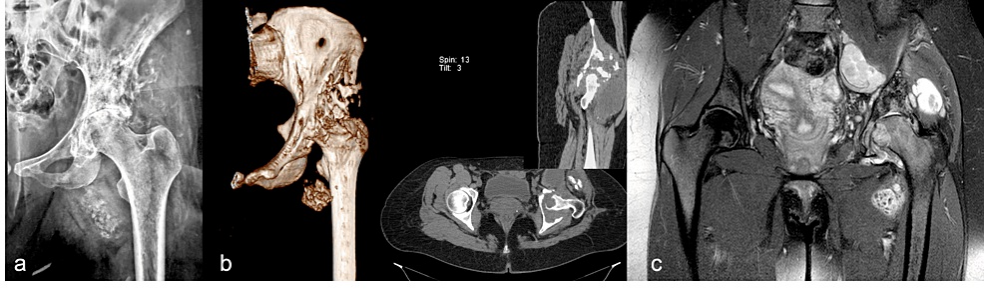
Clinical Case 1: preoperative imaging Preoperative X-ray (a); CT scan showing bone destruction of acetabulum-anterior and posterior walls and iliac (Enneking bone zone Ic+II) of a late-diagnosed patient (b); and preoperative MRI aspect with confluent pseudo-tumoral masses (c).
